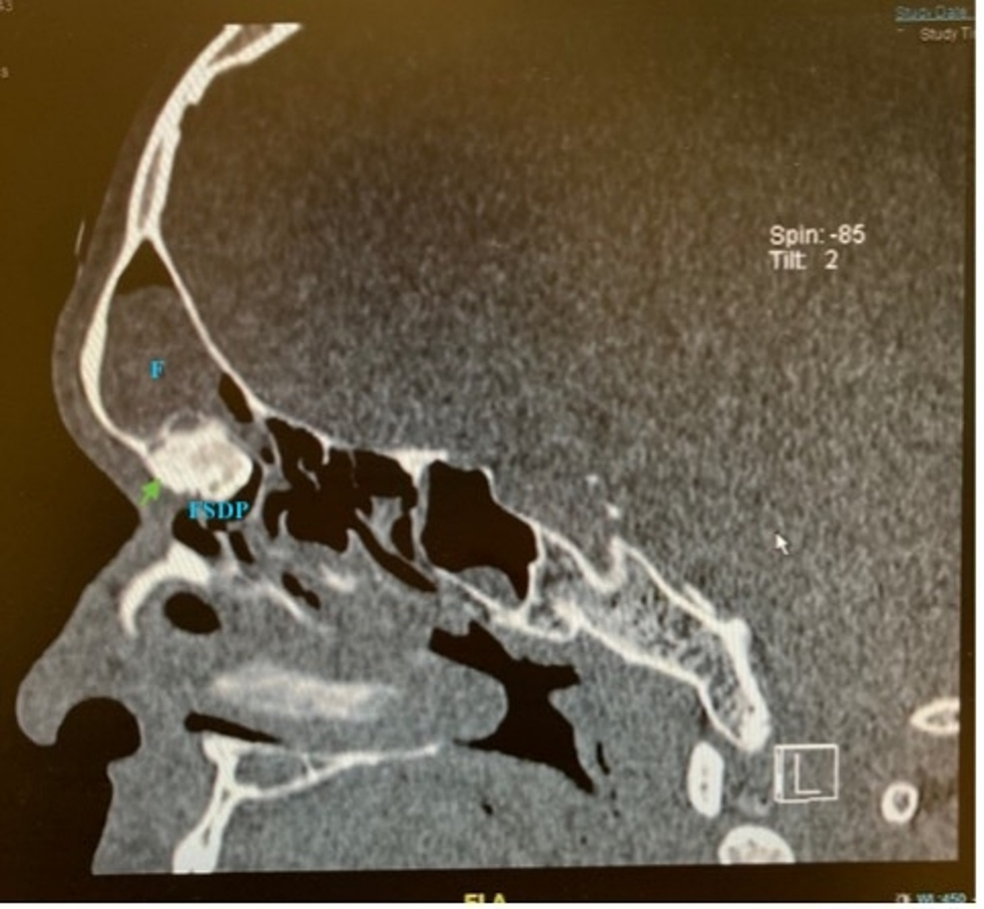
Sagittal view of the patient’s CT PNS The osteoma is indicated by the green arrow. FS: frontal sinus; FSDP: frontal sinus drainage pathway; CT: computed tomography; PNS: paranasal sinus
